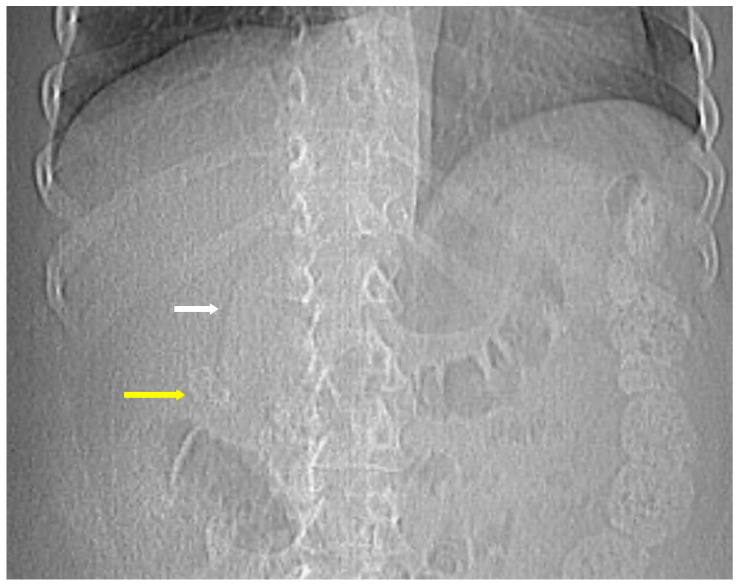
The initial scout image of a computed tomography abdomen and pelvis for a 49-year-old female diabetic patient. Curvilinear lucencies (white arrow) outlining the gallbladder due to emphysematous mural gas within the gallbladder wall and cholelithiasis (yellow arrow) were demonstrated.
